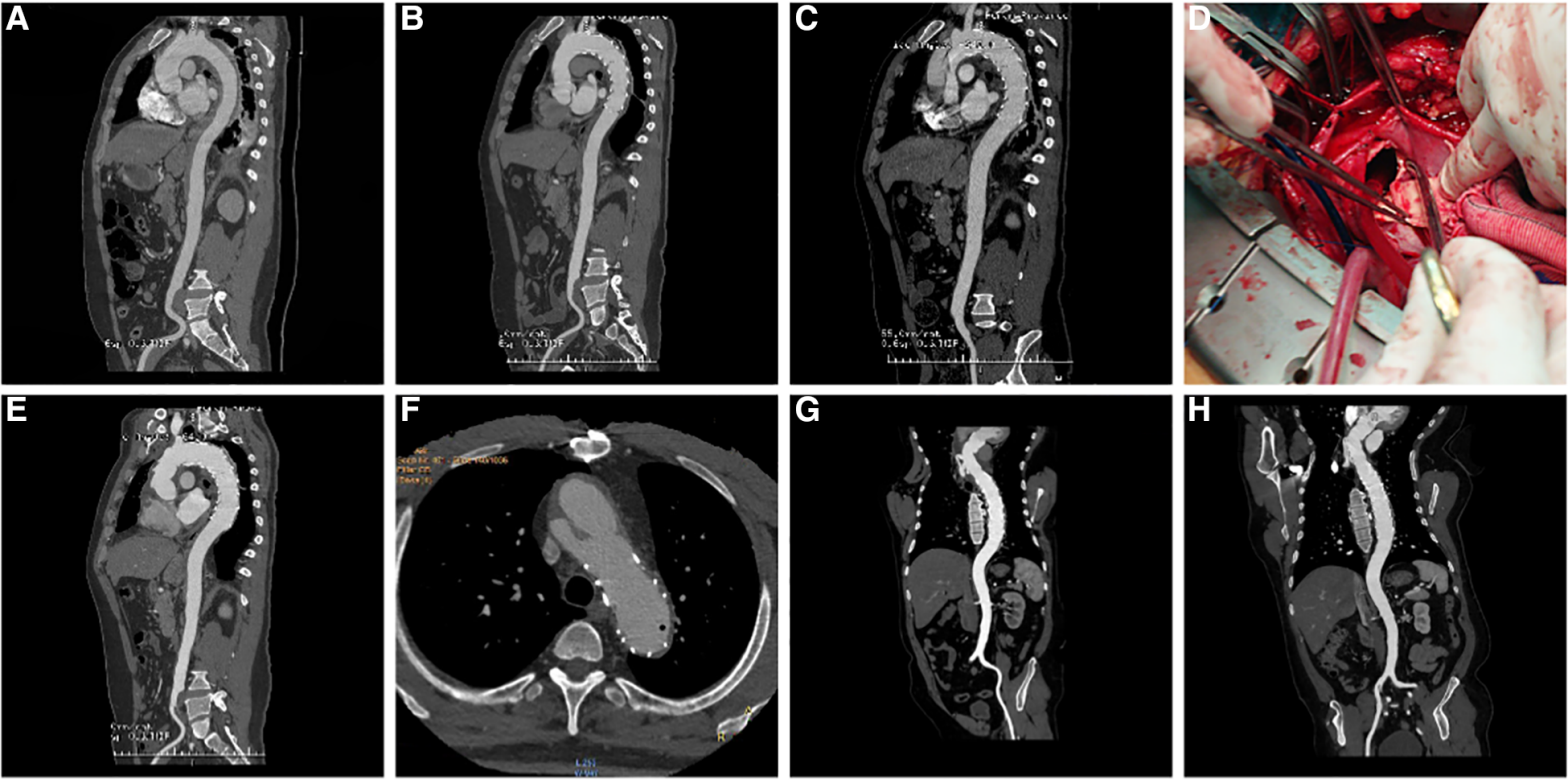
(A) three-dimensional (3D) reconstruction of preoperative surgery CTA showed intramural hematoma of descending aorta; (B) CTA demonstrated that the intramural hematoma was thinner than that before ten days following TEVAR; (C) five weeks following TEVAR, 3D reconstruction of CTA showed RTAD; (D) “ascending aortic replacement, hemi-arch replacement, and stented elephant trunk” was implemented in the emergency, and the entry tear was at the proximal stent; (E) CTA two weeks following the surgery showed changes in the ascending aorta and the arch after replacement; (F) At 90 months after the first surgery, local dissecting aneurysms at the arch were observed; (G) “ascending aortic replacement, total arch replacement, and stented elephant trunk” were performed during the second surgery; (H) CT re-examination on the nine months after second surgery. CTA, CT angiography; TEVAR, Thoracic Endovascular Aortic Repair; RTAD, Retrograde Type A Aortic Dissection; 3D, three dimension.
